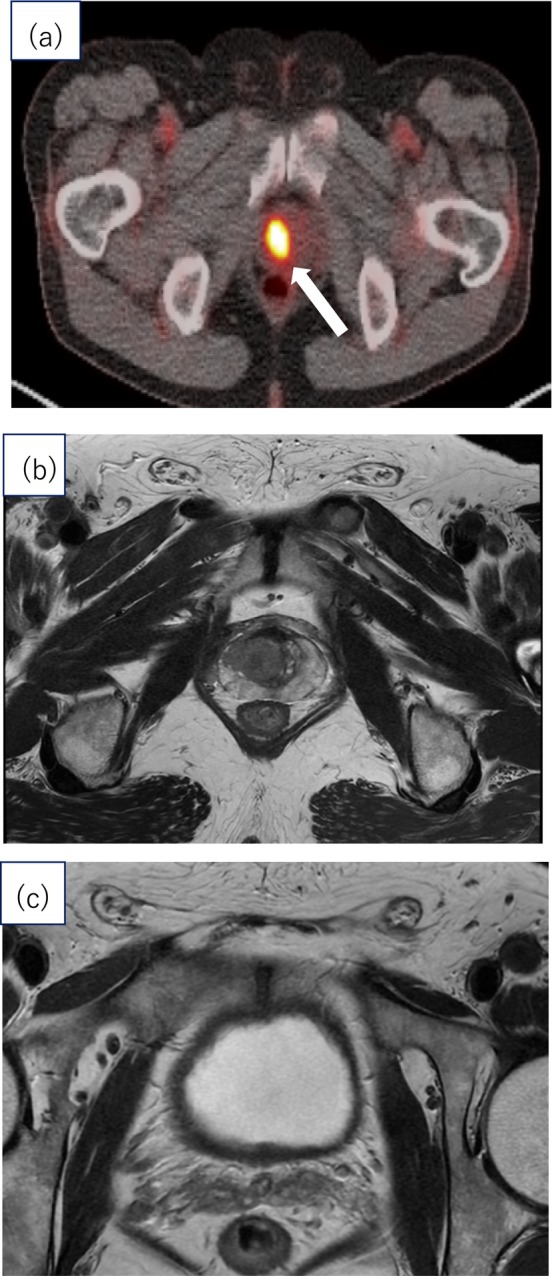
Findings in the Prostate Region: Positron emission tomography‐computed tomography and Magnetic resonance imaging. (A) Abnormal uptake in the right lobe of the prostate on PET‐CT (white arrow). PET‐CT showed no significant FDG uptake in the lymph node region. (b) MRI showed a 15‐mm tumor in the right lobe of the transition zone of the prostate invading beyond the capsule Low signal intensity on T2‐weighted images. (c) MRI revealed no findings suggestive of seminal vesicle invasion, nor were there any findings indicative of pelvic lymph node metastasis on T2‐weighted images.
